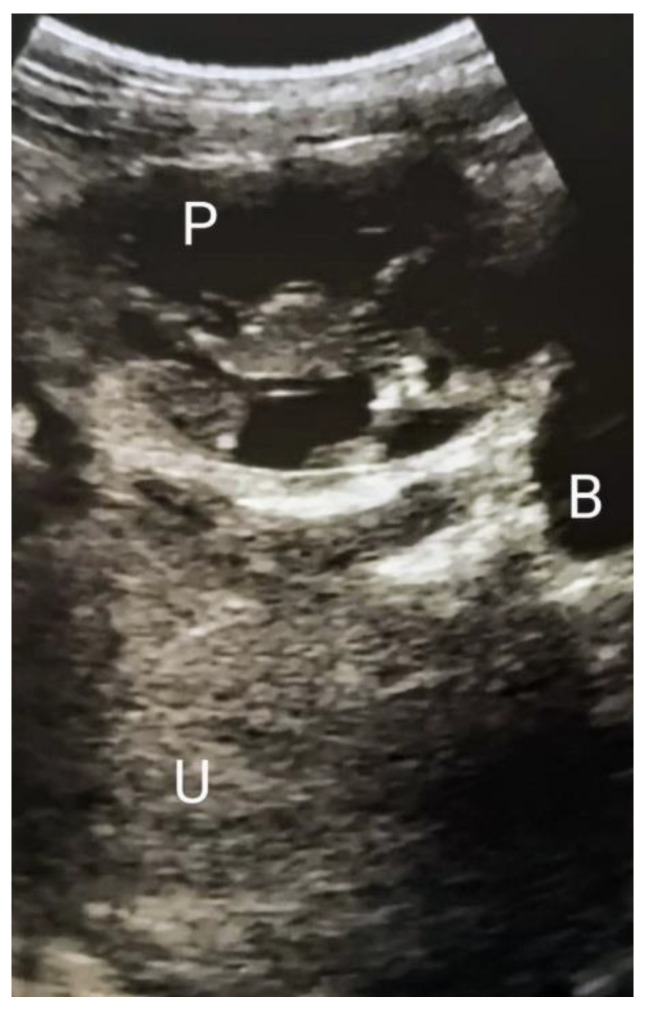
First trimester abdominal ultrasound scan showing the abdominal pregnancy, the empty uterus, and the absence of free fluid in the abdomino–pelvic cavity. P = pregnancy; U = uterus; B = bladder.
